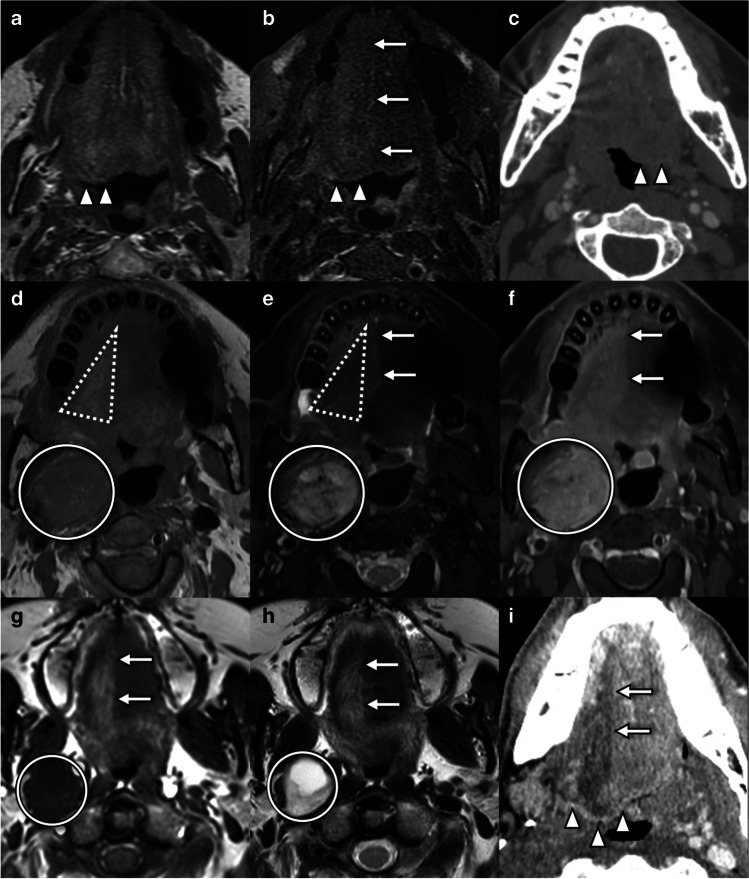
Imaging features of hypoglossal denervation. a Axial T1W sequence in a patient with acute tongue ptosis due to cranio-cervical junction degenerative disease (CCJD) (see Fig. 5) demonstrates posterior displacement of the affected right hemitongue (arrowheads), which remains isointense in signal and bulk. b Axial T2FS sequence in the same patient as a demonstrates posterior displacement (arrowheads) and T2 hyperintensity of the affected right hemitongue. Note the sharply marginated signal change along the median raphe (arrows) which distinguishes this from a mass lesion. c Axial contrast-enhanced CT in a 44-year-old patient presenting with neck pain and tongue deviation demonstrates posterior displacement of the left hemitongue (arrowheads) without change in attenuation. A high cervical carotid dissection was identified (not shown). d Axial T1W sequence in a 29-year-old patient with chronic tongue ptosis due to a jugular paraganglioma (circle). The affected right hemitongue demonstrates volume loss and T1 shortening (dashed triangle) consistent with fatty atrophy. e Axial T2FS sequence in the same patient as d demonstrates signal dropout (dashed triangle) within areas of fatty atrophy, but also some areas of high signal attributed to edema. Note how signal changes respect the median raphe (arrows), distinguishing denervation from a mass lesion. In this case, denervation is caused by extrinsic compression by a vagal paraganglioma (circle) in the carotid space, with characteristic T2 hyperintensity and internal flow voids. f Axial post-gadolinium T1FS sequence in the same patient as d and e demonstrates enhancement of the right hemitongue. Note how signal changes respect the median raphe (arrows), distinguishing denervation from a mass lesion. The vagal paraganglioma (circle) enhances markedly. g Axial T1W sequence in a 54-year-old patient with tongue weakness and episodic dizziness demonstrates T1 shortening and volume loss in the right hemitongue respecting the median raphe (arrows) compatible with fatty atrophy. In this case, denervation is caused by extrinsic compression by a T1 hypointense schwannoma (circle). h Axial T2W sequence in the same patient as g demonstrates T2 prolongation and volume loss in the right hemitongue respecting the median raphe (arrows) compatible with fatty atrophy. The causative schwannoma (circle) is of intermediate T2 signal with a T2 hyperintense cystic component. i Axial CT in a patient with chronic tongue ptosis due to osseous metastasis (not shown) demonstrates posterior displacement (arrowheads), hypoattenuation, and volume loss in the right hemitongue respecting the median raphe (arrows) compatible with fatty atrophy
